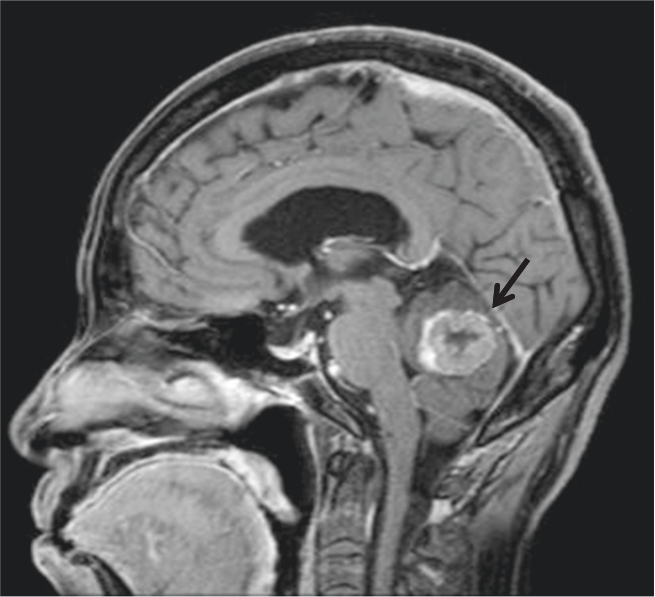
Sagittal view T1-weighted magnetic resonance imaging (fat suppression) of the brain shows a 2.5-cm tumor with surrounding edematous change (arrow).
